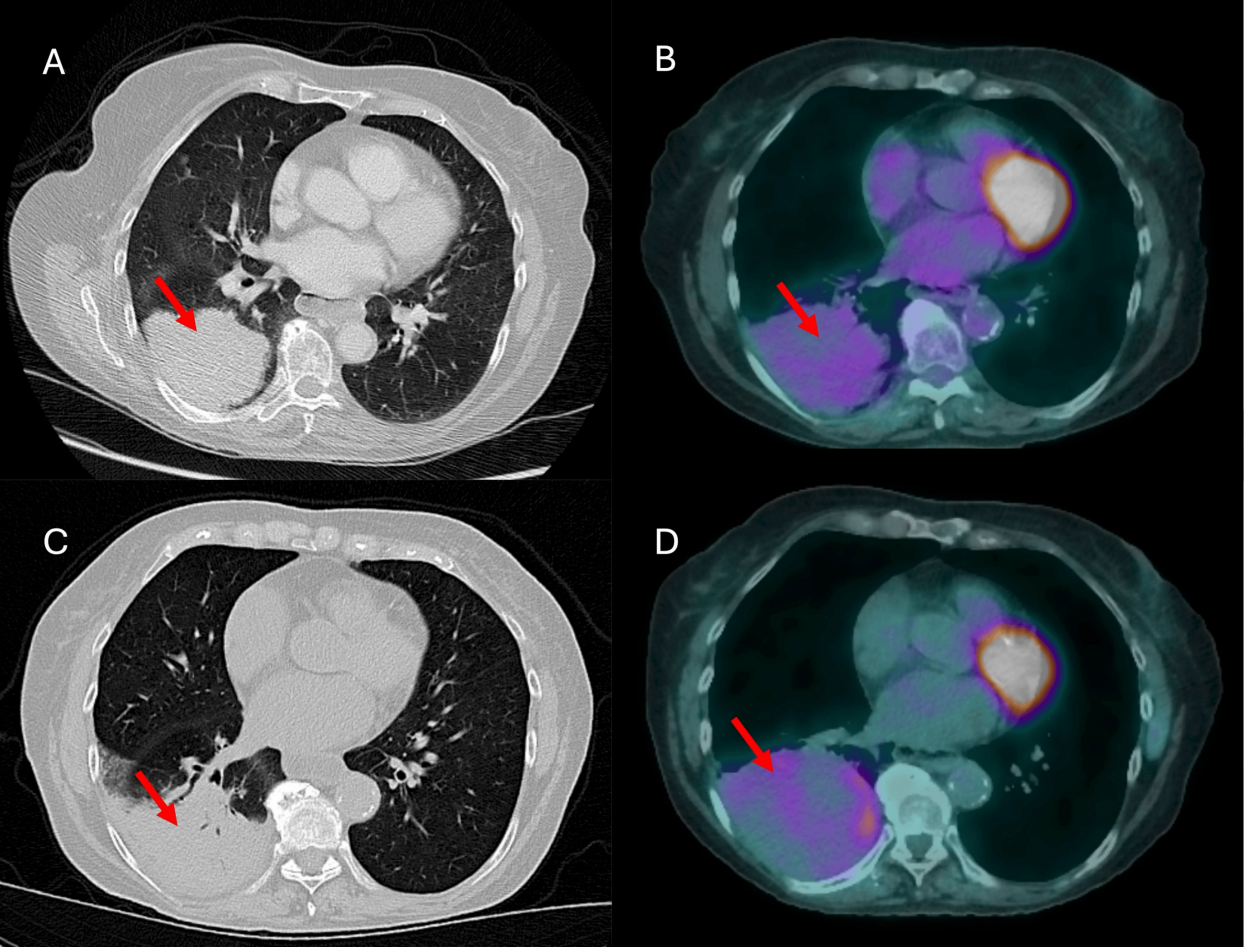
CT and PET/CT imaging (A) CT of the right lower lobe lung cancer (red arrow) and (B) PET/CT of the cancer before induction of chemoimmunotherapy.  (C) CT and (D) PET/CT images of the right lower lobe cancer after chemoimmunotherapy.
